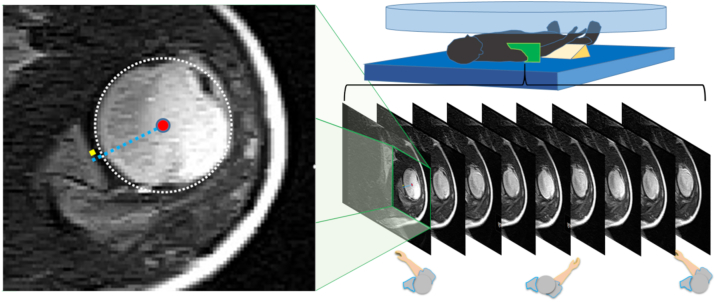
MRI scanning sequence and data analysis. Participant position during active shoulder rotation in the MRI scanner (*Right* figure) and measurement of the humeral head position (*Left* figure). The center of the humeral head was calculated using the least-squares method based on the coordinates traced on the articular surface. The *light blue line* projects the center point of the humeral head vertically onto the glenoid fossa. The *yellow dots* indicate the midpoint of the glenoid. Humeral head deviation was measured as the distance between the *light blue line* and the *yellow dot* on the glenoid fossa. *MRI*, magnetic resonance imaging.
